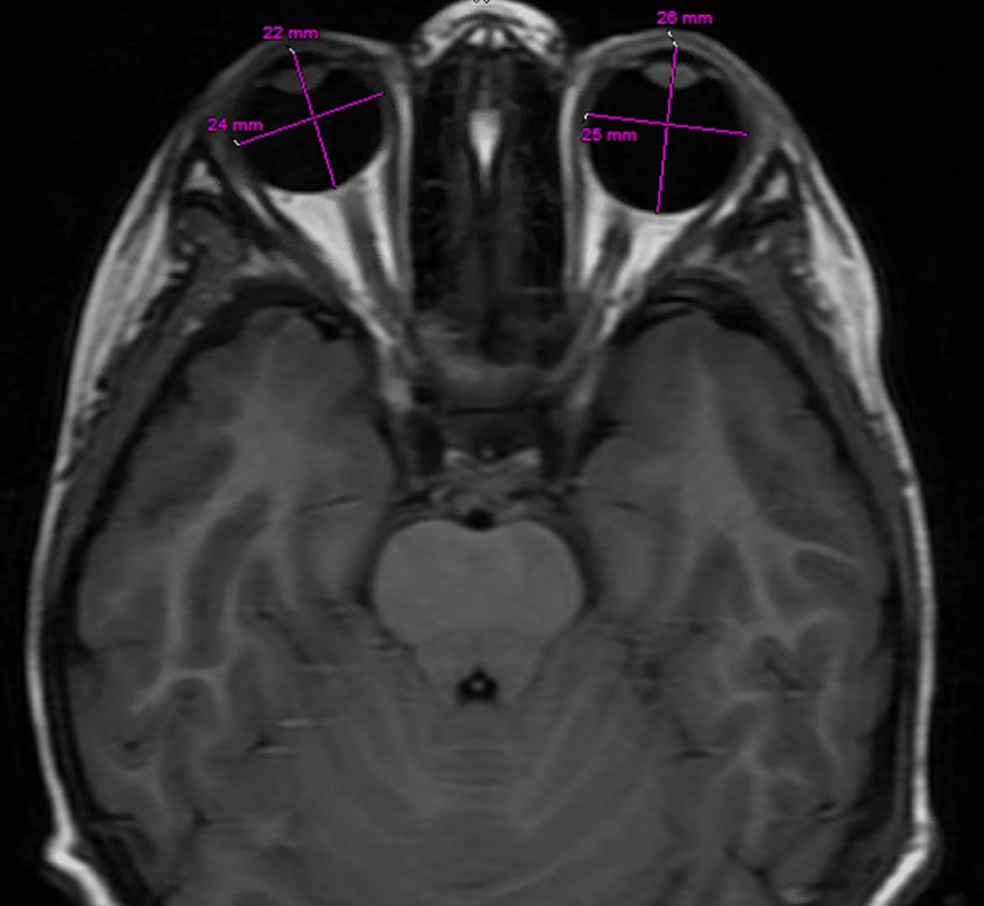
Baseline magnetic resonance imaging of the Brain T1 FLAIR from 2020 showing asymmetric axial elongation to 26 mm of the left eye, compared to 22 mm in the right eye.
